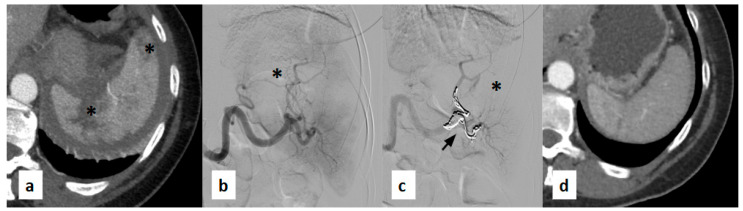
(a) Contrast-enhanced axial CT scan acquired during the arterial phase shows lacerated-contused areas with extensive full-thickness multilinear laceration extending from the hilum (black asterisks). (b) Digital subtraction angiography (DSA) performed using a 5F Cobra-shaped catheter (Cordis, FL, USA) confirms the full-thickness hilar laceration of the spleen (black asterisk) and altered intraparenchymal vascularization previously identified on CT. (c) Post-procedural DSA performed through a guiding catheter demonstrates embolization of the branches feeding the hypervascular areas using Penumbra coils (Crossmed, Turin, Italy) (black arrow), resulting in marked reduction of vascularization of the splenic parenchyma (black asterisk). (d) Follow-up contrast CT scan reconstruction shows partial recovery of splenic volume with an almost homogeneous contrast enhancement of the residual splenic parenchyma.
